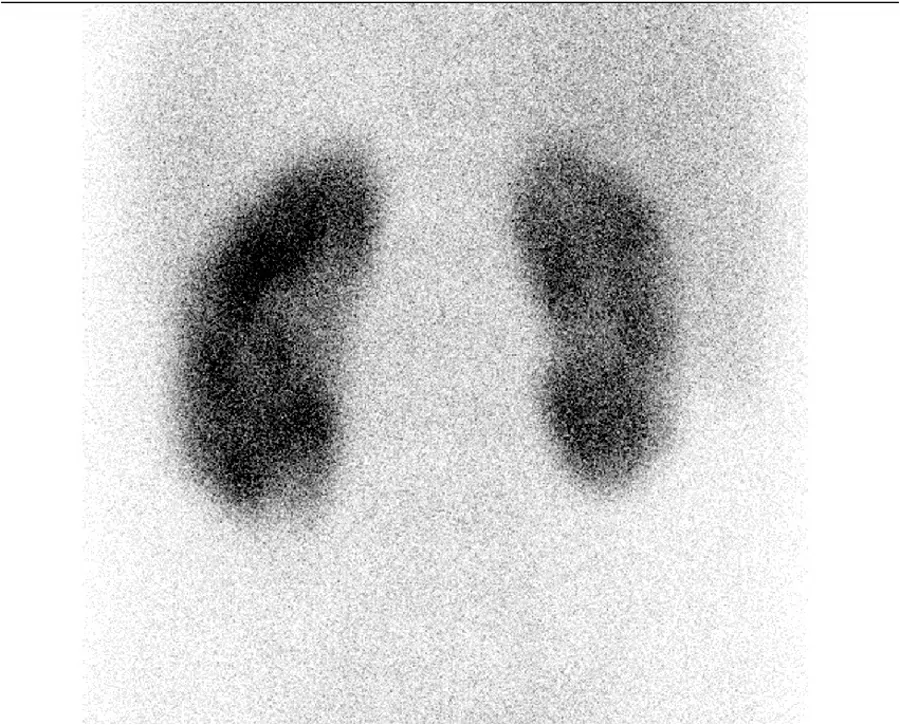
Renal static scintigraphy findings. Both kidneys are in normal position with regular contours. The right kidney is normal in size, while the left kidney shows a slight increase in size. Radiotracer distribution within both kidneys is heterogeneous, with multiple areas of decreased tracer uptake. A markedly reduced tracer distribution is noted in the left renal pelvic region. Impression: Bilateral pyelonephritis is highly suspected. Concomitant renal cortical scarring cannot be excluded, and left renal pelvic hydronephrosis is present.
